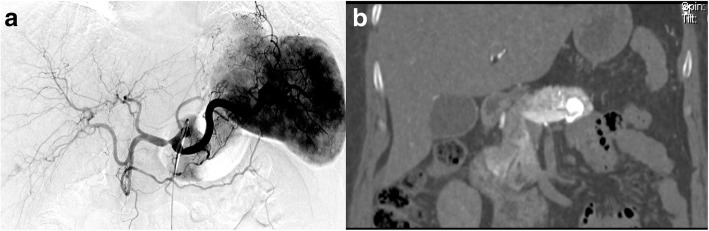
A 55-year old female. a Celiac artery angiography does not show any lesion. b CT during celiac artery angiography indicates a suspected lesion in the pancreas tail. Lesion is considered to overlay with spleen
